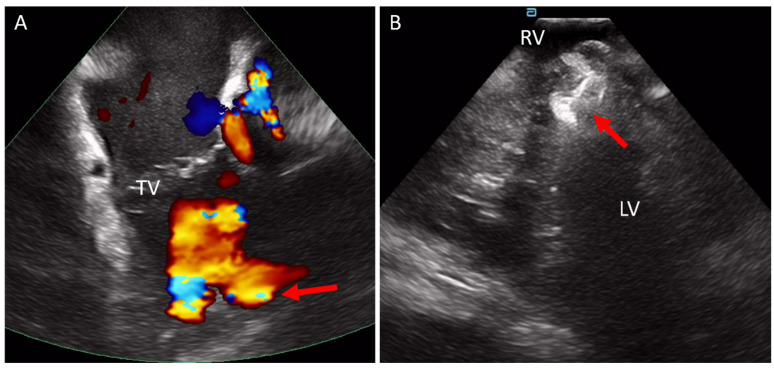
ICE catheter in the right atrium. (A) Color Doppler from the right atrium, looking through the tricuspid valve (TV) at the right ventricle and visualizing color flow through the muscular septum consistent with a muscular VSD (arrow). (B) ICE catheter within the RV. The muscular VSD occlude seen after deployment. ICE, intracardiac echo; LV, left ventricle; TV, tricuspid valve; VSD, ventricular septal defect.
